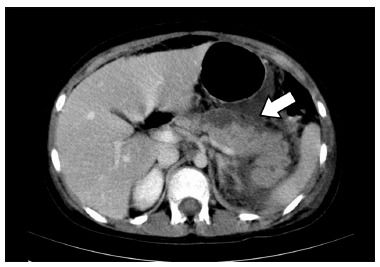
Interstitial edematous pancreatitis: CT exam in axial T2 cut
